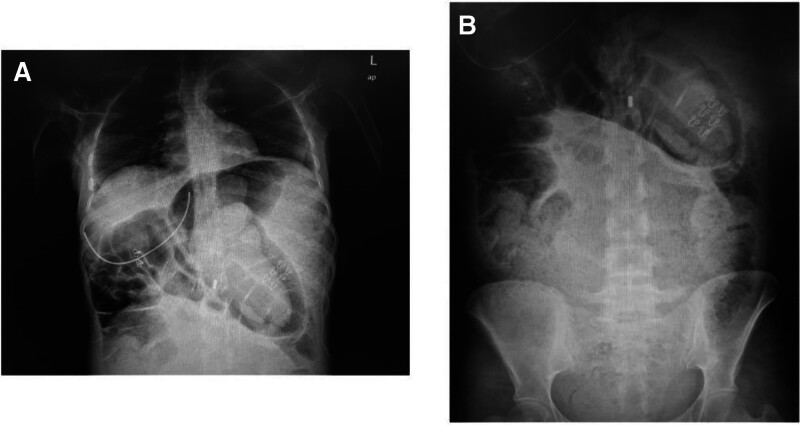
Abdominal X-ray at presentation demonstrating features of toxic megacolon. (A) Abdominal X-ray revealing marked colonic dilation with a loss of haustrations, highly suggestive of toxic megacolon. No evidence of free air to indicate perforation. (B) Significant colonic dilation is evident, with associated fecal impaction. A nasogastric tube is visible in the upper abdomen. These findings further support the diagnosis of toxic megacolon with intestinal obstruction.
